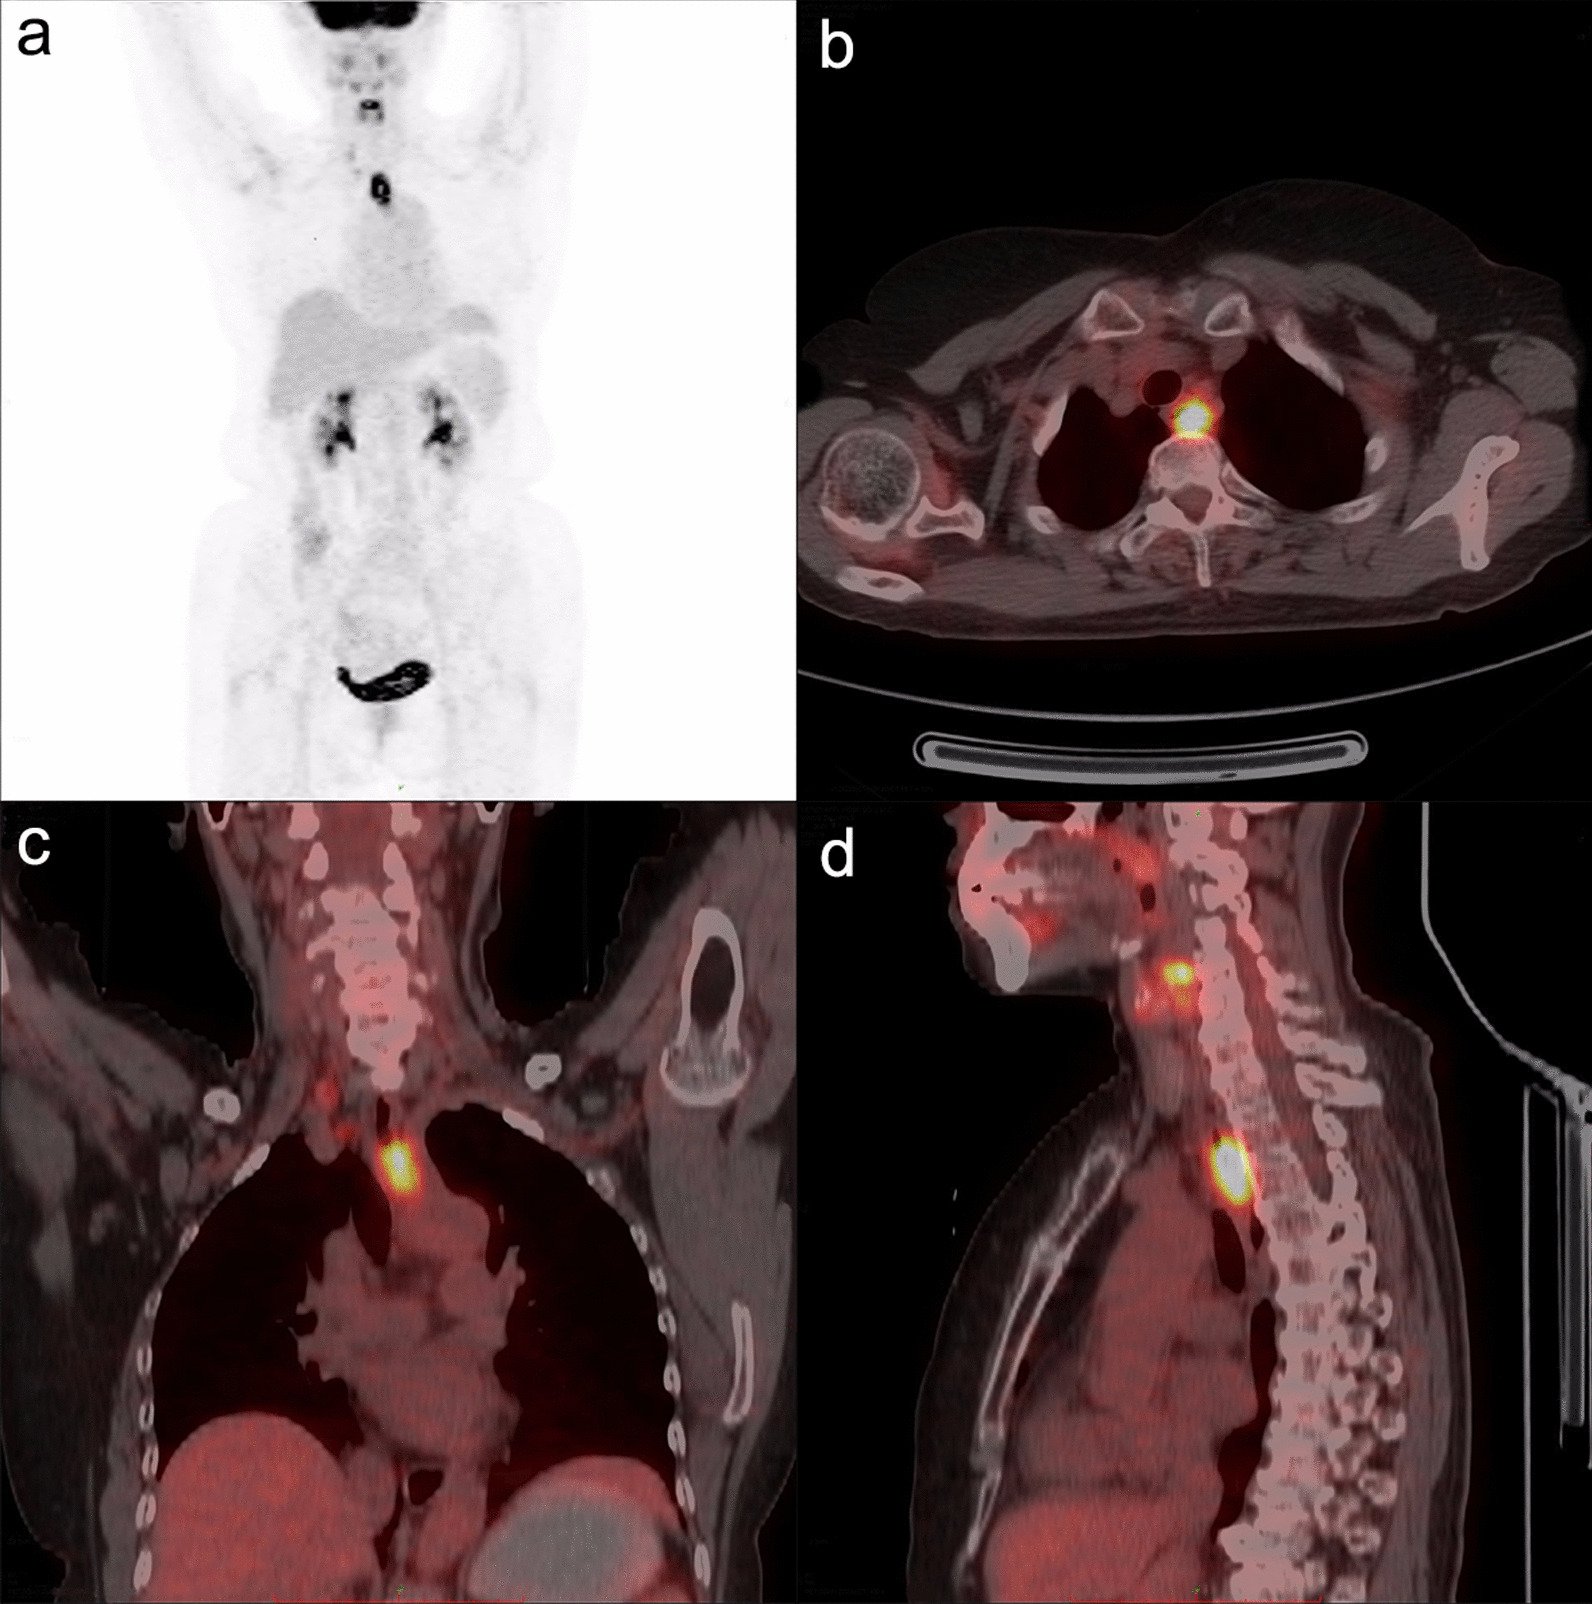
A 68-year-old female with esophageal squamous cell carcinoma. The ^18^F-FDG PET/CT fusion image showed thickening of the upper esophageal wall characterized by increased metabolism. SUV_Tumor_, MTV and TLG were 21.7, 4.69cm^3^ and 57.3 g, respectively. Hypermetabolic right cervical root and right paratracheal lymph nodes, consistent with metastases, were detected. The PLNS number was 2, SUV_LN_ and SD_max(LN-T)_ were 3.75 and 0.155 m^−1^, respectively. The patient received definitive chemoradiotherapy and was alive at the end of follow-up with an OS of 28 months
